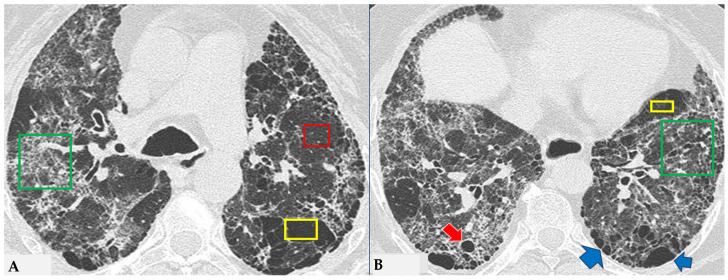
FHP: chest HRCT ((A,B): axial scan). Visible is the “three density pattern”, with areas of the lung with normal density spared from pathological changes (red box); areas of the lung with reduced density characterized by a paucity of vascular and bronchial structures (yellow box); areas of the lung with increased density showing reticulations, interstitial thickening and traction bronchiectasis (green box). Lung cyst (red arrow) and areas of smoking-related paraseptal emphysema and advanced destructive emphysema (ADE) (blue arrows) are also present.
